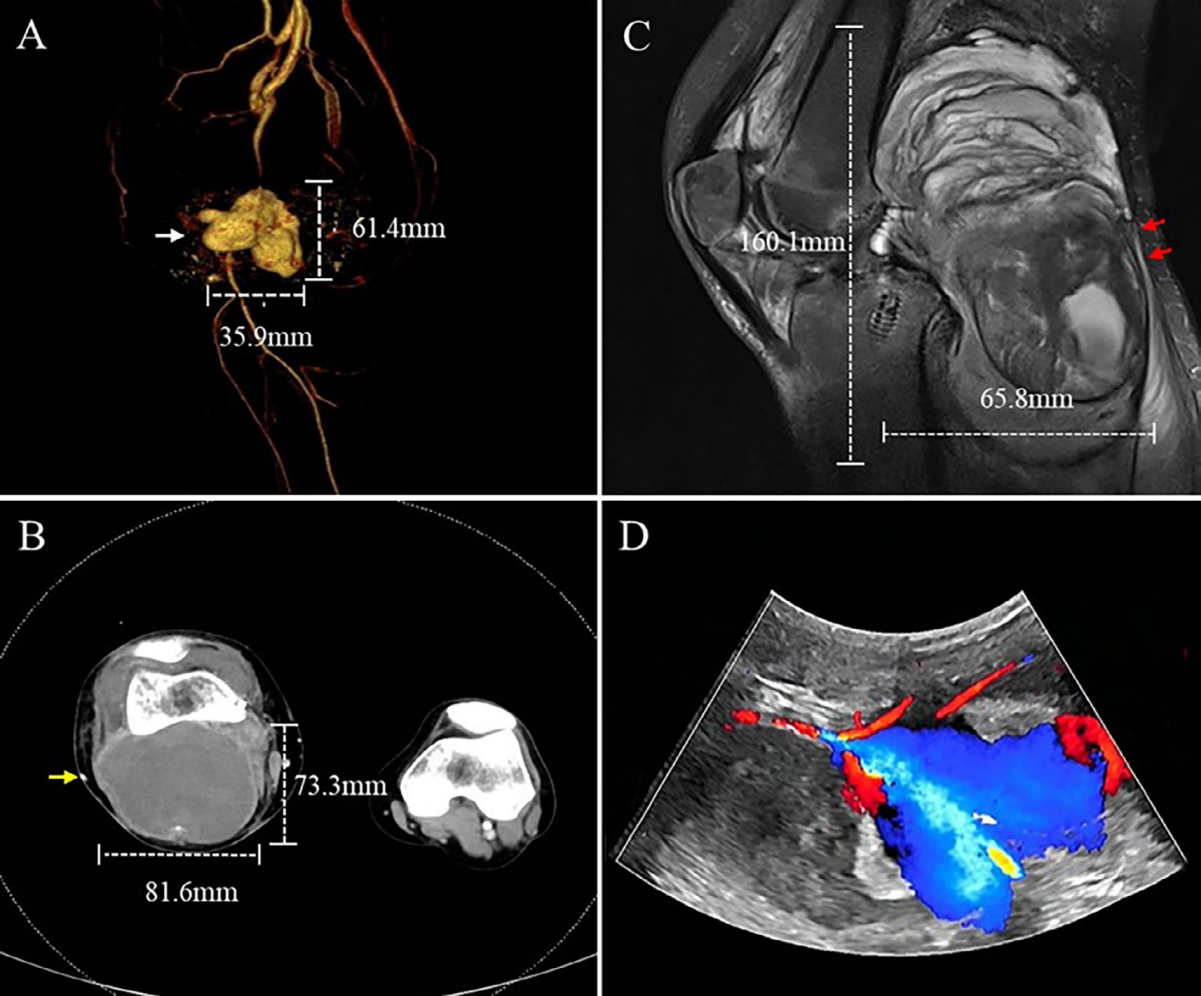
Preoperative imaging results of the patient. (A) Preoperative CTA revealed a popliteal mass measuring approximately 61.4 × 35.9 mm (white arrow). (B) An axial view of the preoperative CTA showed an occupancy of approximately 73.3 × 81.6 mm (yellow arrow). (C) Preoperative MRI showed a clear‐cut mass‐like mixed signal in the popliteal fossa with a cross‐sectional area of approximately 160.1 × 65.8 mm (red arrow). The signal intensity of the PCLR graft is mixed. (D) Color Doppler ultrasound of the lower extremity vessels showed a mixed echogenic mass in the popliteal fossa.
